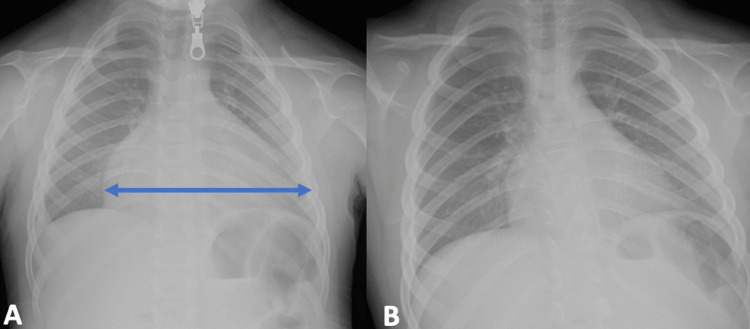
(A) Chest radiograph showing cardiomegaly with globular enlargement of the cardiac silhouette. (B) Chest radiograph, taken after six months, revealing no evidence of cardiomegaly.
